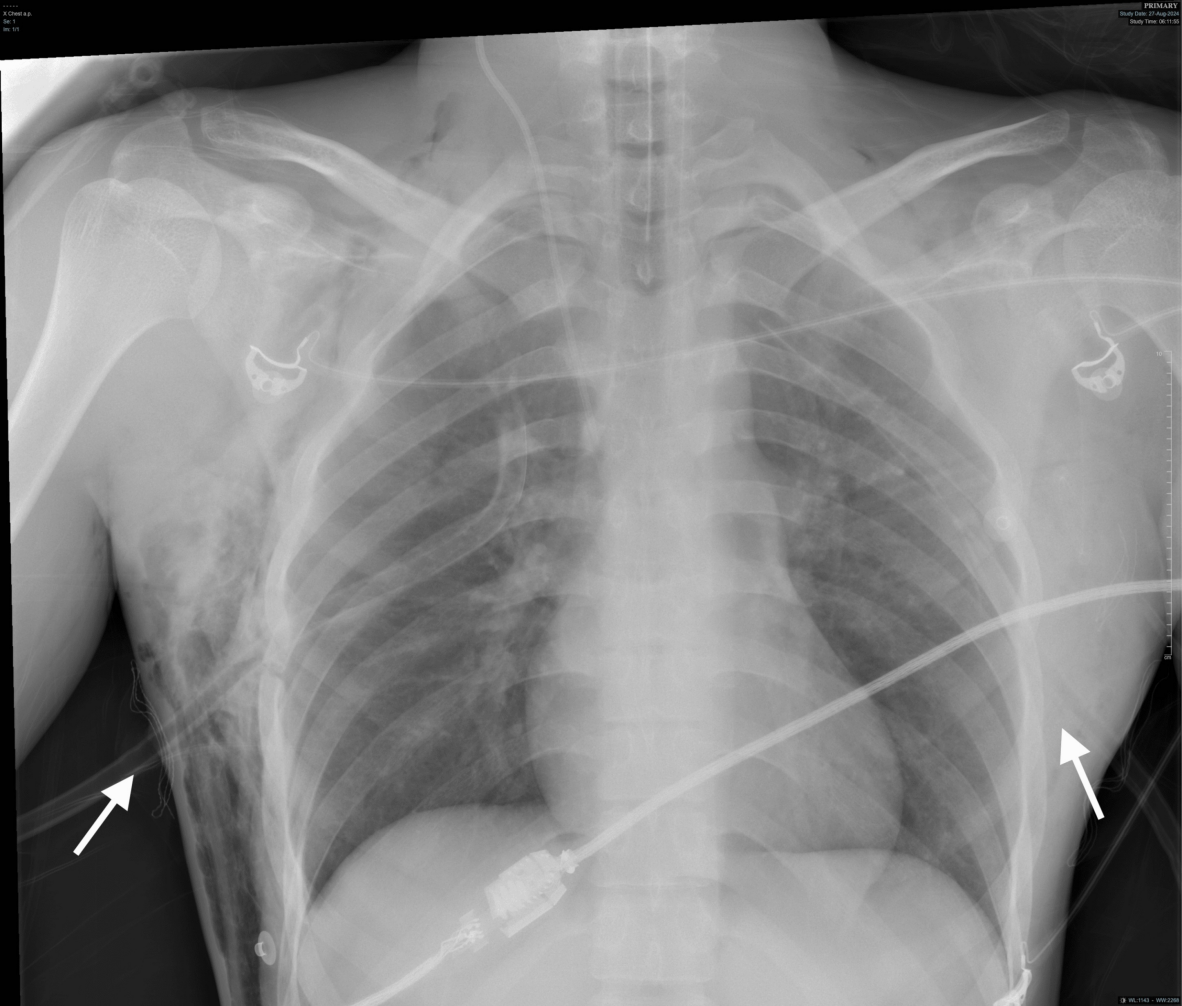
Chest X-ray showing the presence of bilateral intercostal drainage tubes (white arrows)
